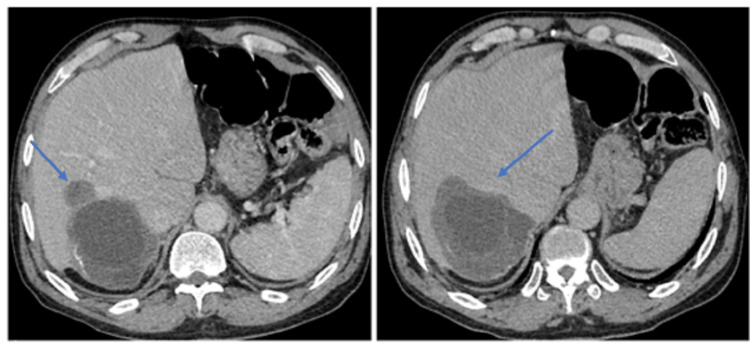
Axial abdominal CT showing hepatic hydatid cyst CT: computed tomography
